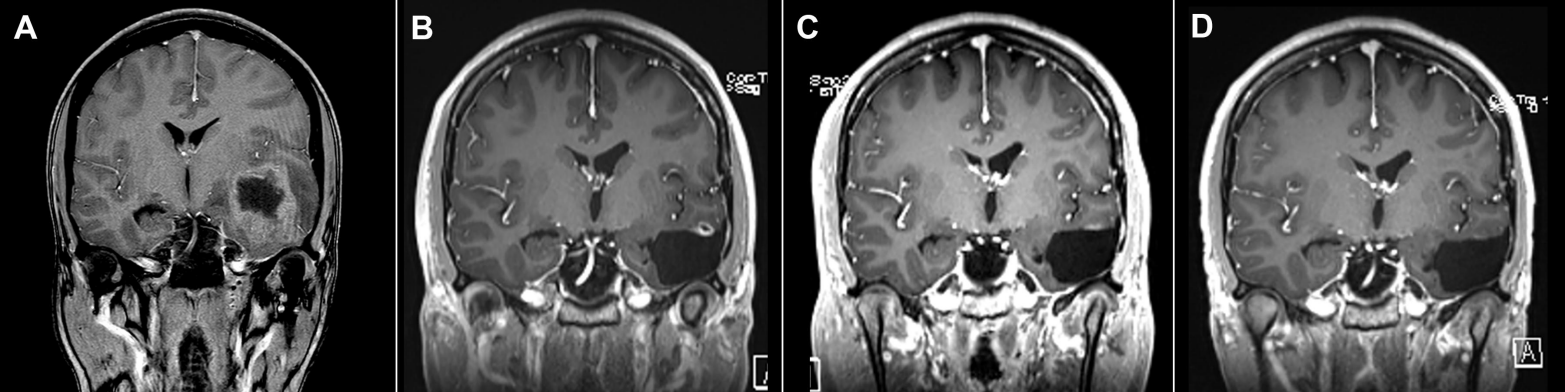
26-year-old female (A) November 2017, 1.5 Tesla scanner: T1-weighted postcontrast gradient echo preoperative image showing GBM in the left temporal region. (B) April 2019, T1-weighted postcontrast gradient echo image showing long-lasting postoperative enhancement suspicious of residual tumor. (C) August 2019, T1-weighted postcontrast gradient echo image reveals a decrease in enhancement. (D) March 2022, T1-weighted postcontrast gradient echo image with no signs of tumor recurrence.
